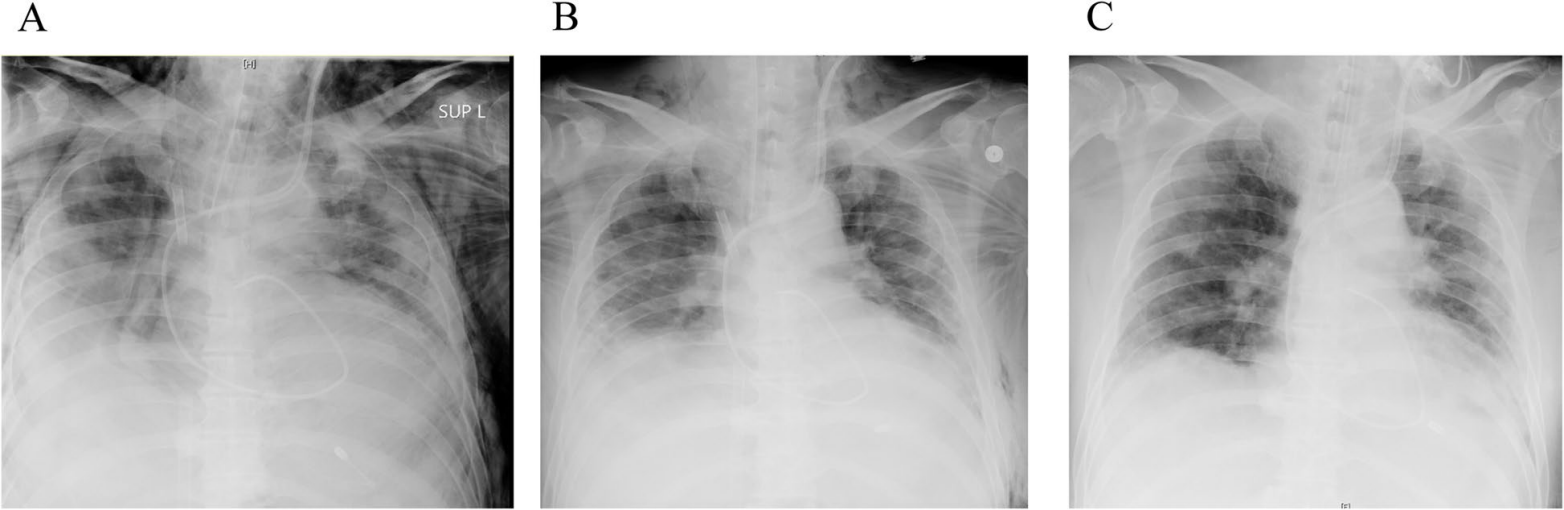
Chest X-ray of the patient. A On admission. B Fourth day post-ECMO cannulation. C Post-ECMO decannulation. These X-rays showed that extensive pneumomediastinum/subcutaneous emphysema gradually decreased
